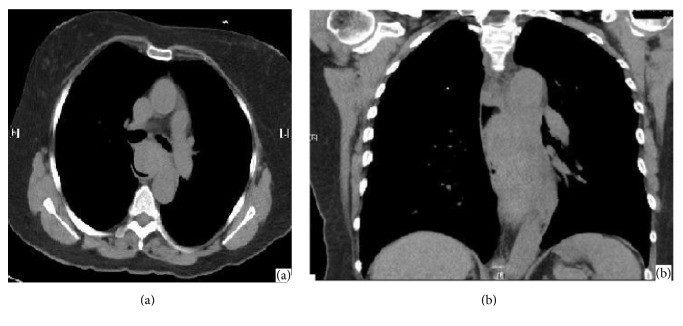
Initial CT demonstrating the long intramural oesophageal haematoma ((a) sagital and (b) coronal view).
